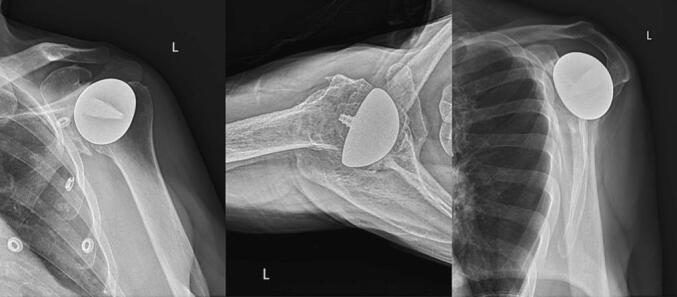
10-year postoperative radiographic imaging of iTSA in patient II.
